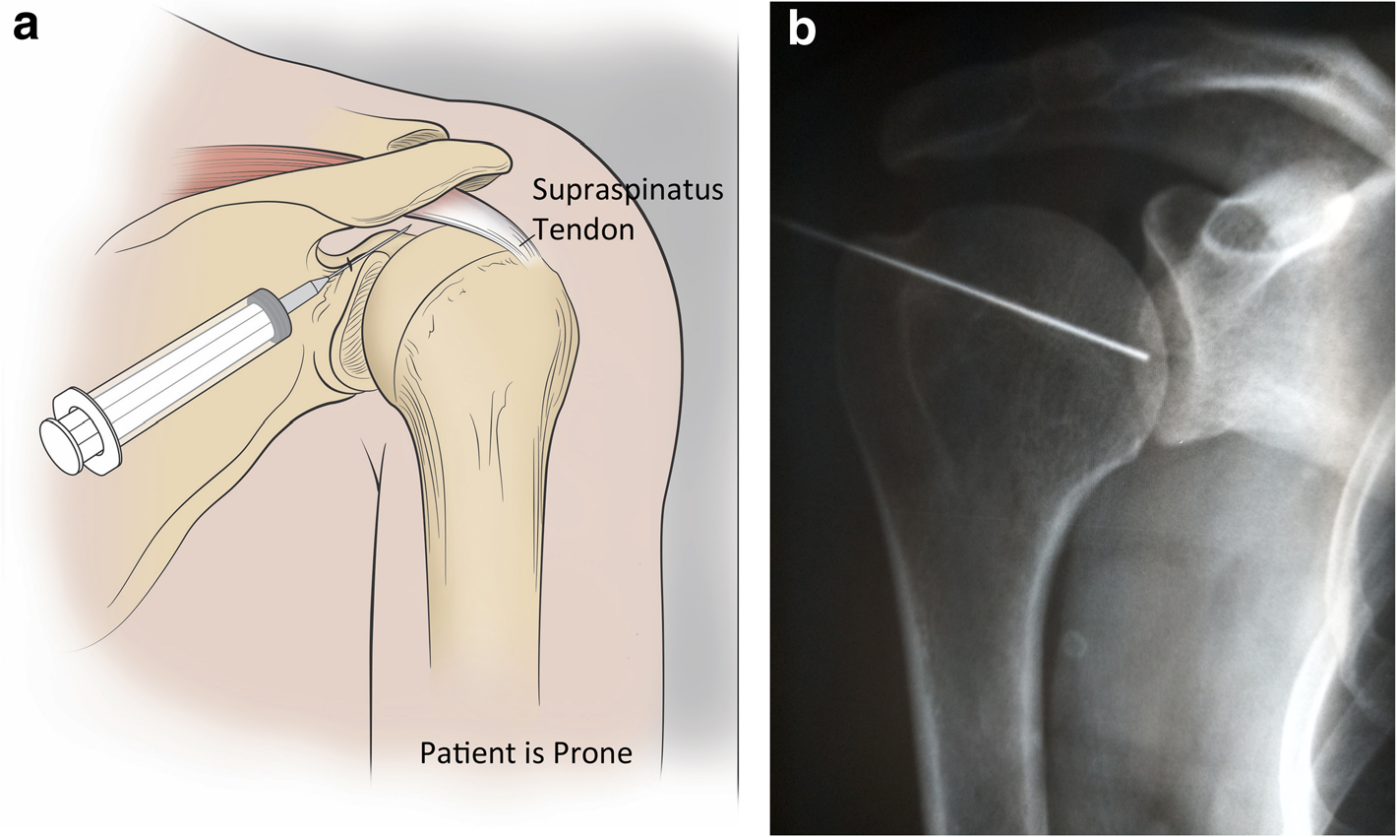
a Second injection was made into the glenohumeral intra-articular space under the supraspinatus tendon at or just proximal to the superior equator of the head while patient was prone. b X-ray image shows needle placement for the second injection
